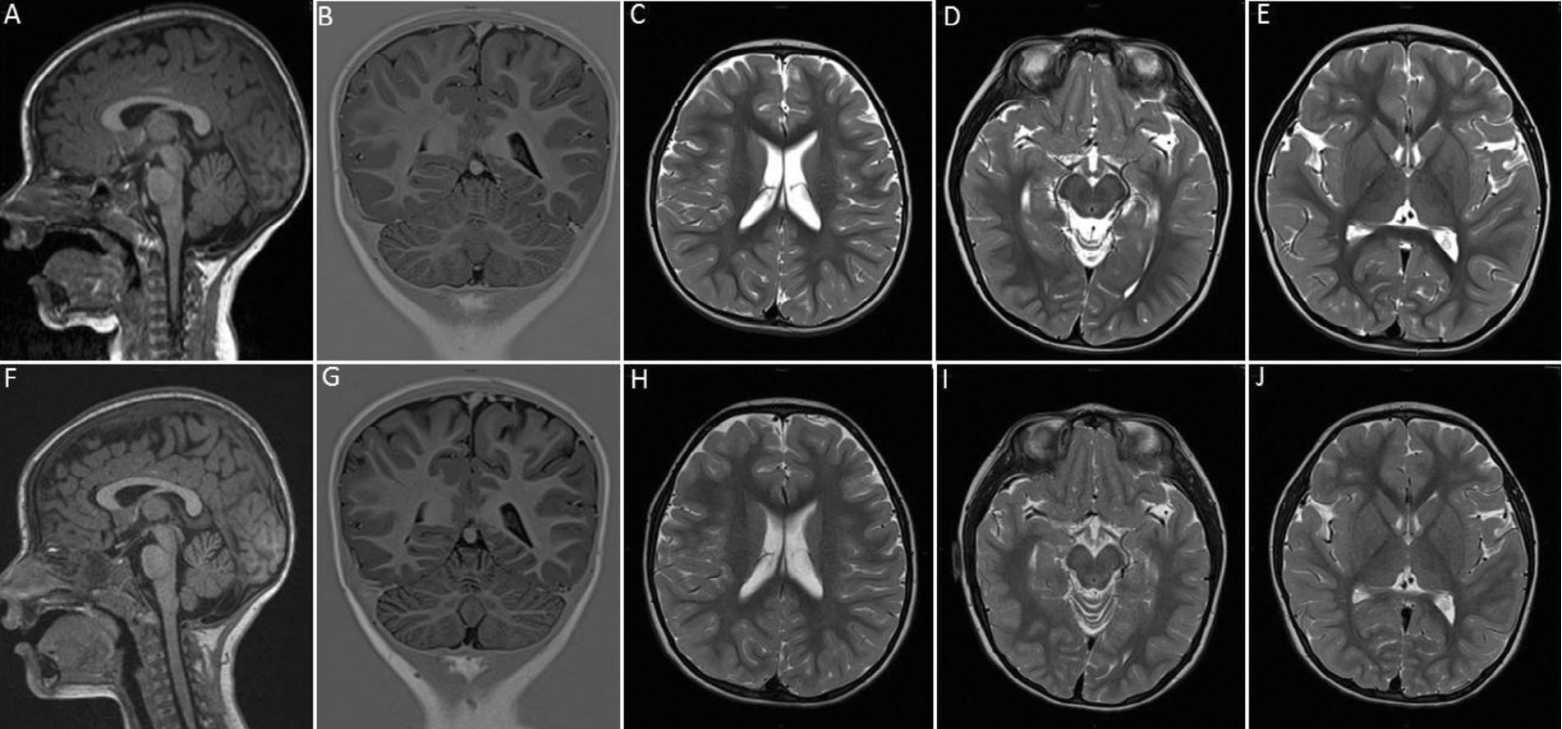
Brain MRI at 2.4 years of age shows mild atrophy of the superior cerebellar vermis (A), while the cerebellar hemispheres, white matter, mesencephalon and basal ganglia are normal (B–E). Follow‐up brain MRI at 3.4 years reveals progression of the cerebellar atrophy to involve the hemispheres (F, G), while the white matter, mesencephalon and basal ganglia are still normal (H–J).
